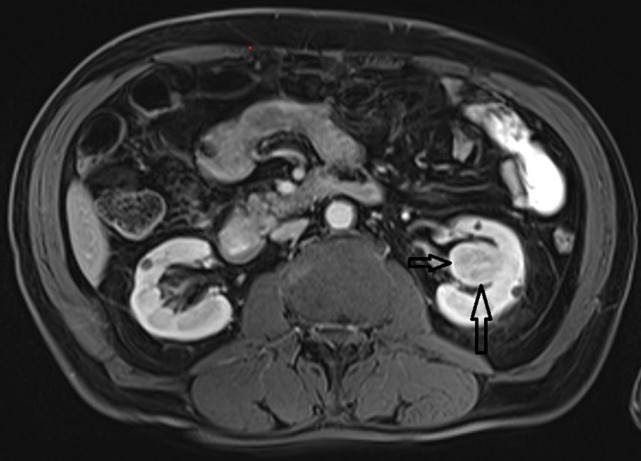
contrast enhancement in the left renal mass by magnetic resonance imaging
